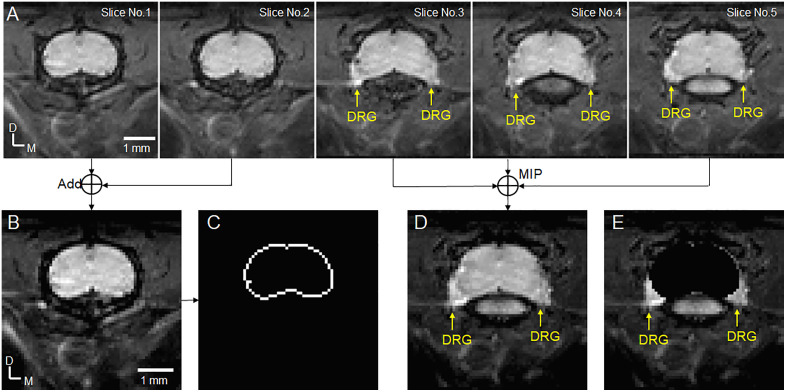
DRG delineation. (A) images from 5 consecutive axial slices (slice thickness = 0.29 mm). The DRGs are observed in slices No. 3, 4 and 5, but not in slices No. 1 and 2. (B) MRI image of spinal cord without DRGs by averaging slice No. 1 and 2. (C) Spinal cord edge from the spinal cord image in (B). (D) MRI image of the spinal cord with DRGs from maximum intensity projection (MIP) of slice No. 3, 4 and 5. (E) DRG image from (D) by zeroing the spinal cord region defined in (C). D: dorsal, M: medial.
